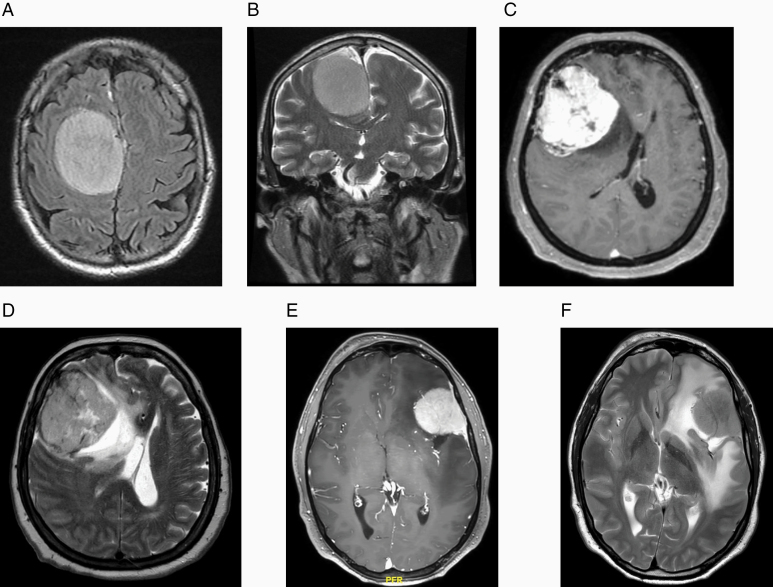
Peritumoral edema was classified according to Trittmacher criteria: grade 0 (A, axial Flair sequence and B, coronal T2-weighted sequence); grade 1 (C, axial T1-weighted sequence with gadolinium and D, axial T2-weighted sequence); grade 2 (E, axial T1-weighted sequence with gadolinium and F, axial T2-weighted sequence).
